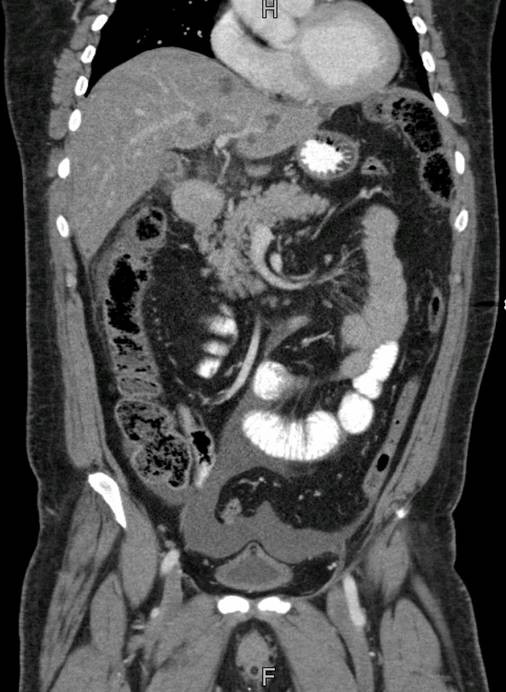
Sagittal section of abdominal CT scan showing pericholecystic fat stranding, and mild fluid in the pelvis.
